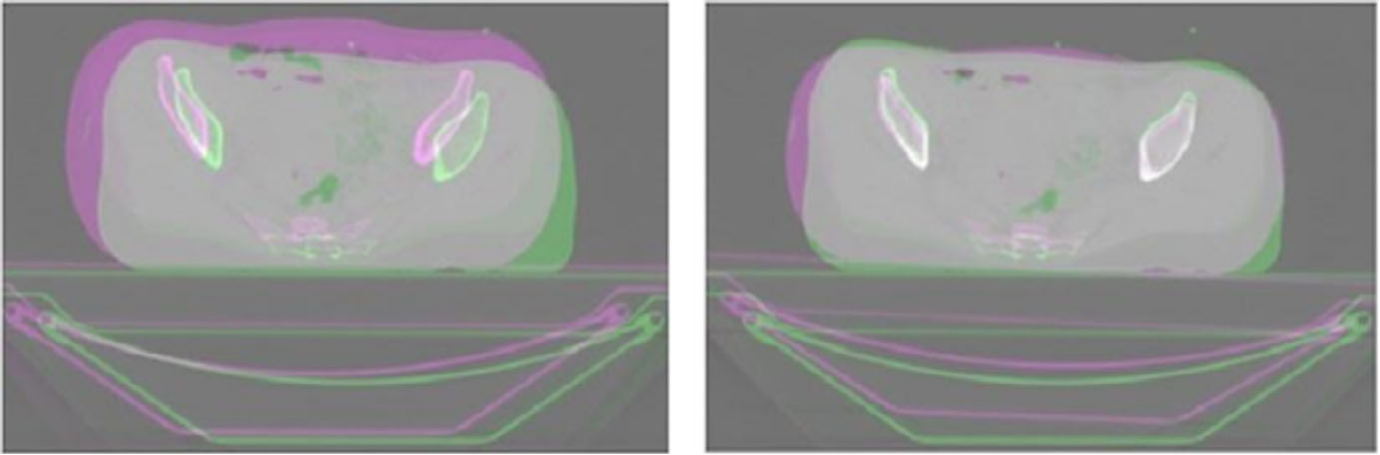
Difference between CT studies of patient no. 1 before registration (left) and after affine registration (right).
